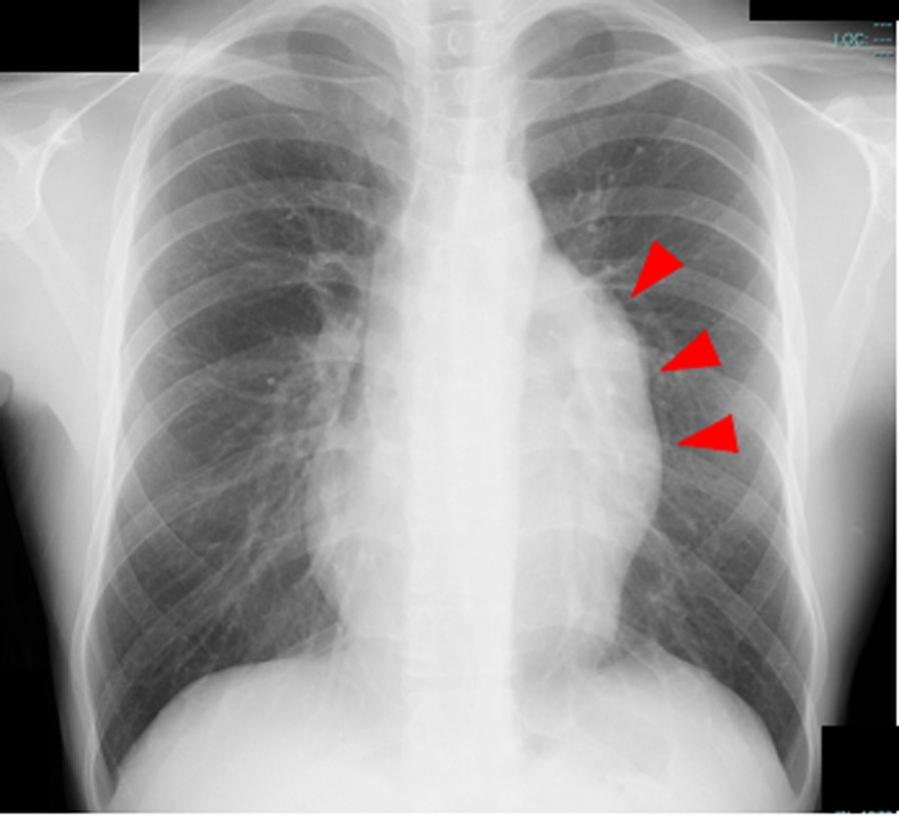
Pre-operative chest X-ray showed an abnormal mass in the left hilum region (arrowheads)
